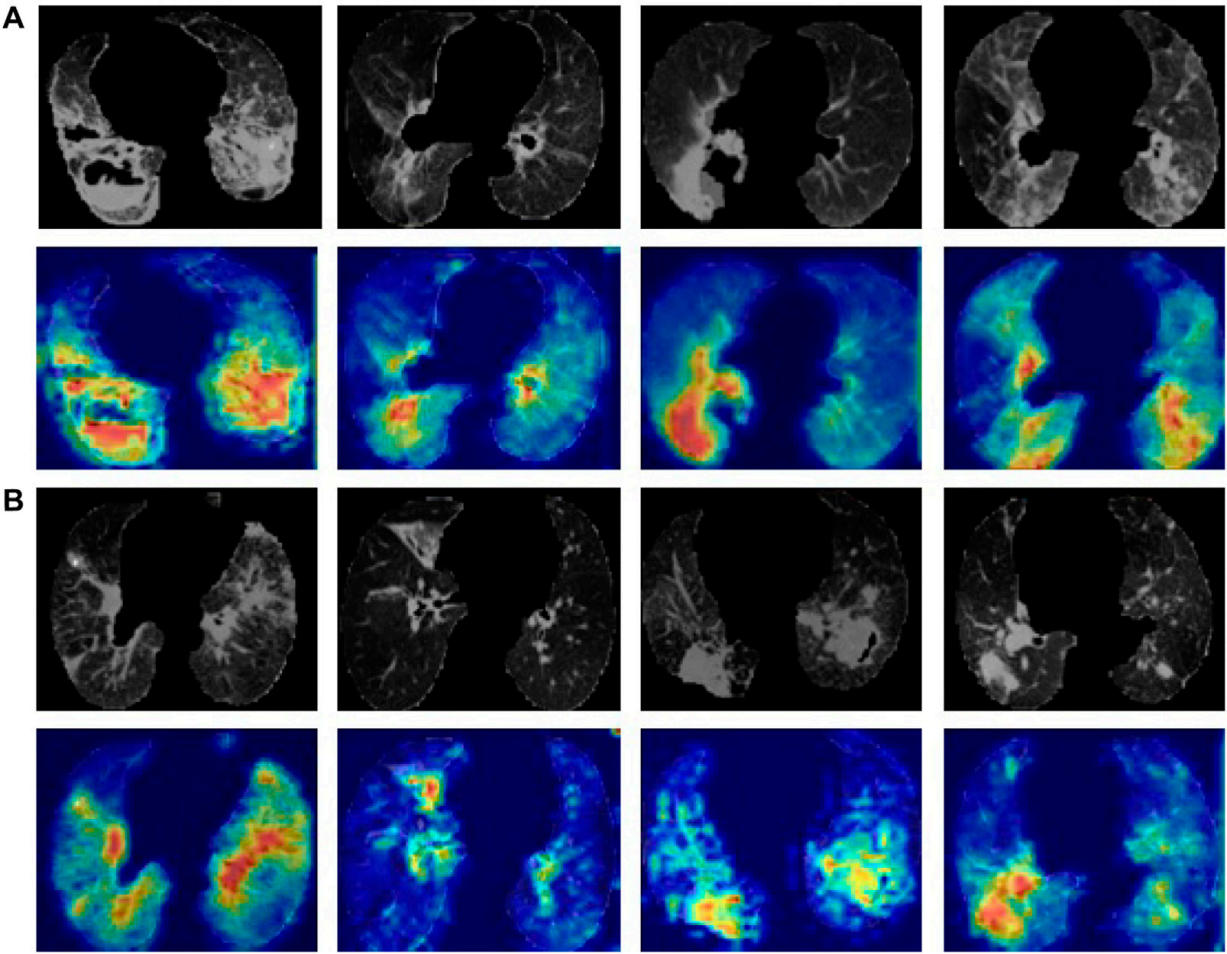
Lung CT images and the corresponding CAMs. (A) ATB CT images of the lung field and the corresponding CAMs. (B) Non-ATB CT images of the lung field and the corresponding CAMs.
